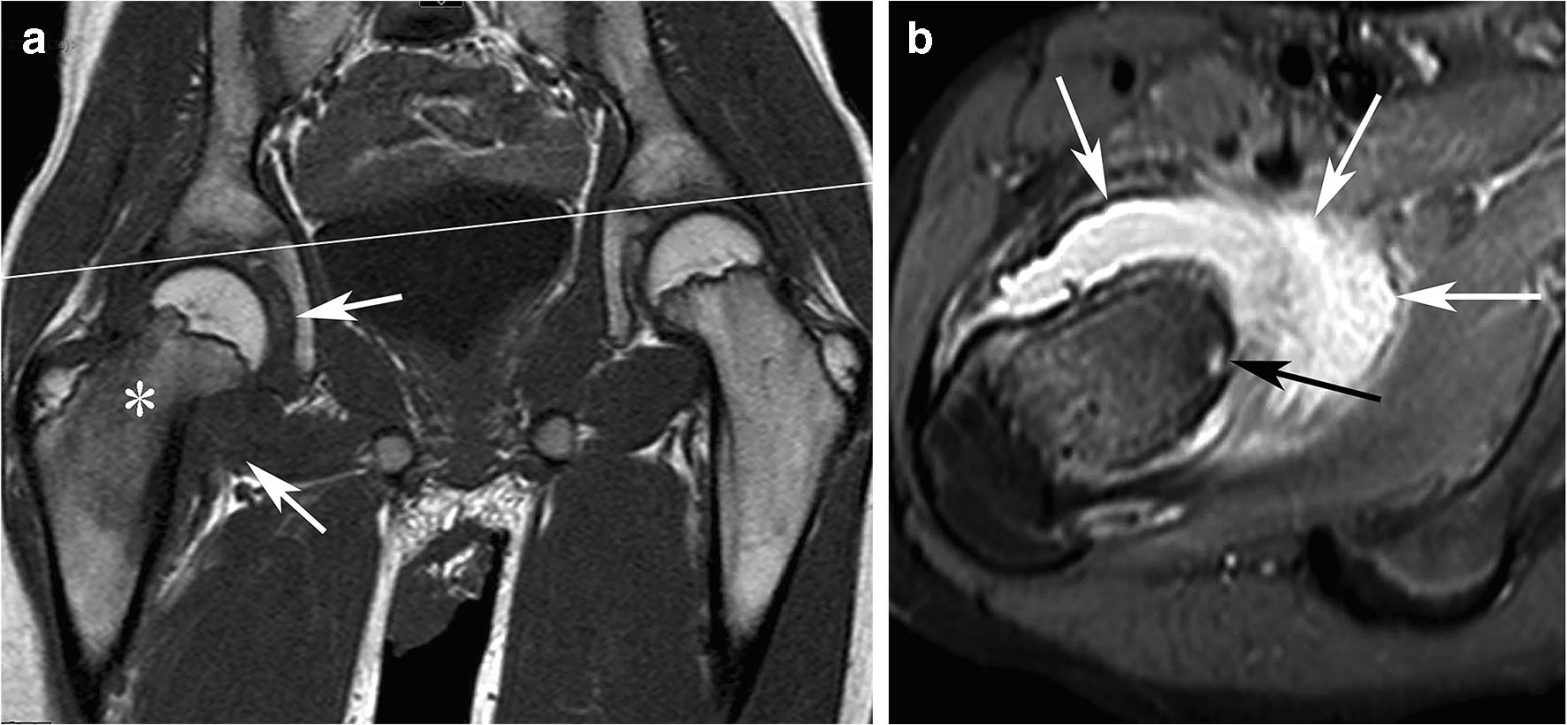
Painless OO in a 13-year-old boy with a painless limp and normal spinal MRI (not shown) requested for the limping gait. A coronal T1-weighted image of the pelvis (a) shows abduction of the right femur relative to the pelvic plane (line), low signal intensity in the bone marrow of the right femoral neck (asterisk), and synovial swelling with low signal intensity (arrows). An axial fat-saturated proton density-weighted MR image (b) shows both synovial swelling with high signal intensity (white arrows) and the OO nidus in the femoral neck (black arrow), which was confirmed by a CT scan (not shown). The OO was then successfully treated by radiofrequency ablation
